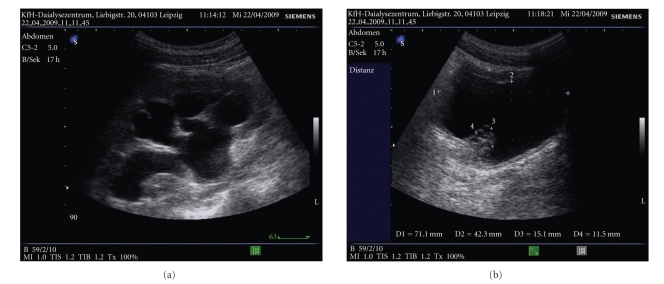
Ultrasound examination of the right kidney and the bladder on admission. Hydronephrosis stage III-IV with severely reduced renal parenchyma (a) and bladder tumorous formation (b).
